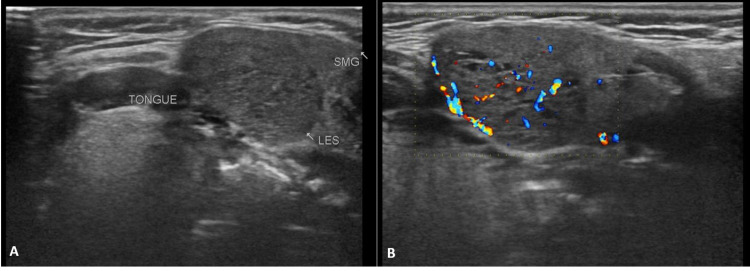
Ultrasound (USG) of the left submandibular region shows a well-defined, round to oval, heterogeneous isoechoic lesion compressing the submandibular gland with few cystic foci within (A) and increased vascularity on color Doppler (B).
